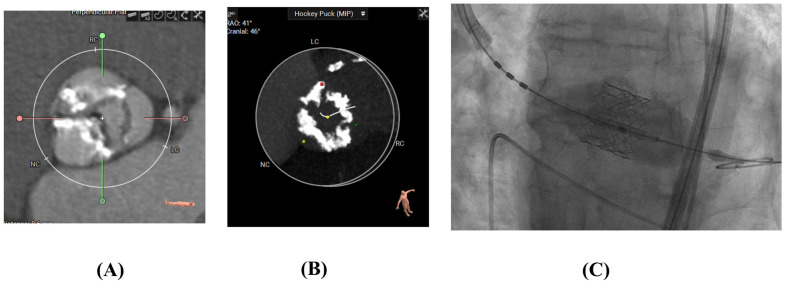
(A,B) CT scans of calcified aortic valves pre-TAVR (C). Edwards-Sapien TAVR Valve Deployed.
